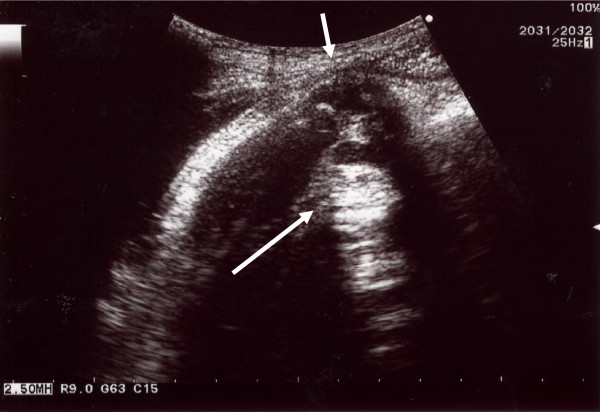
An abdominal ultrasound image of the uterine wall and the fetal minor part. The small arrow indicates a thin uterine wall, which is slightly bulging. Beneath the thin uterine wall a fetal minor part (large arrow) is visible, which was palpated as a hard mass through the abdomen.
